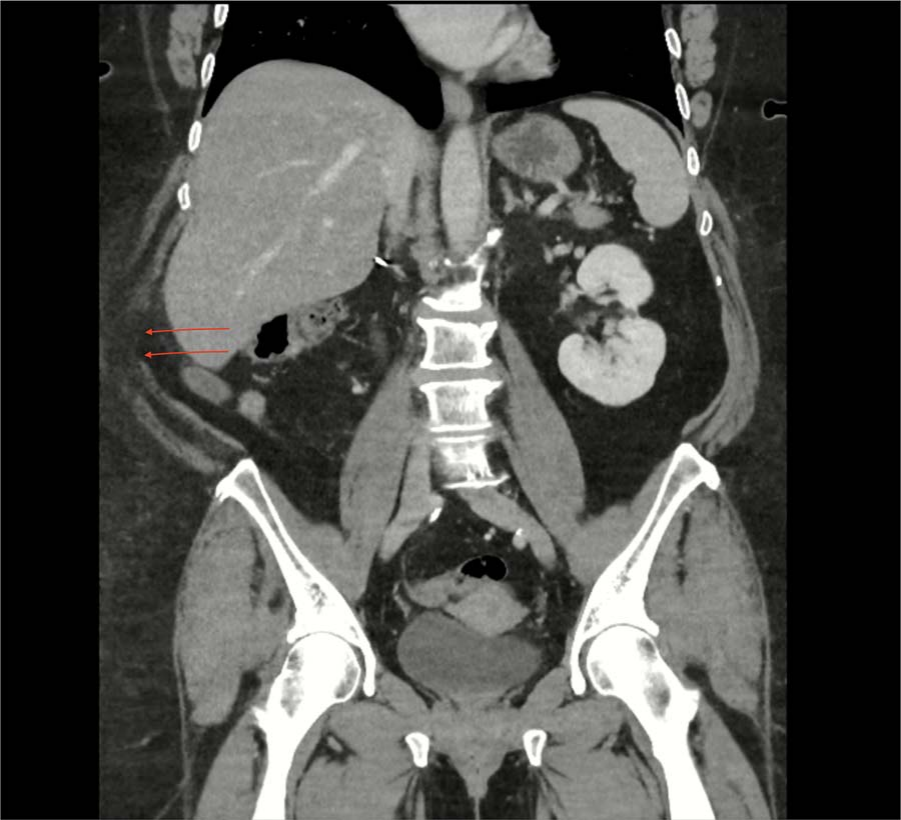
CT coronal section highlighting notable right posterolateral abdominal wall defect (arrows).
